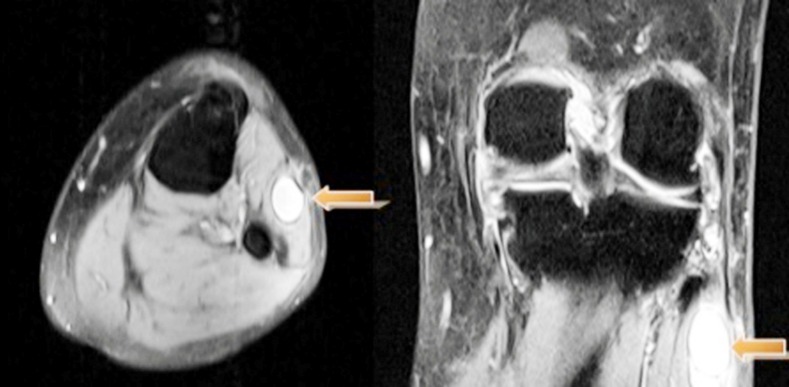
Proton density-weighted fat-saturated axial and coronal magnetic resonance images show close relationship of the cystic lesion with the peroneal nerve.
